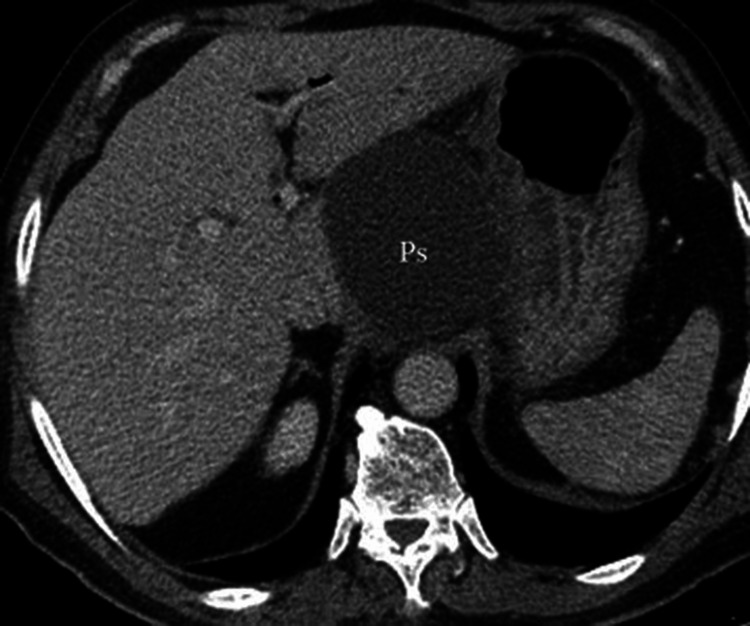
Preoperative contrast-enhanced computed tomography (CECT) abdomen (axial section) revealing a giant pancreatic pseudocyst (Ps) in the lesser sac measuring in the region of the body and tail of pancreas, with no internal debris or enhancing components, for which cystogastrostomy was planned
